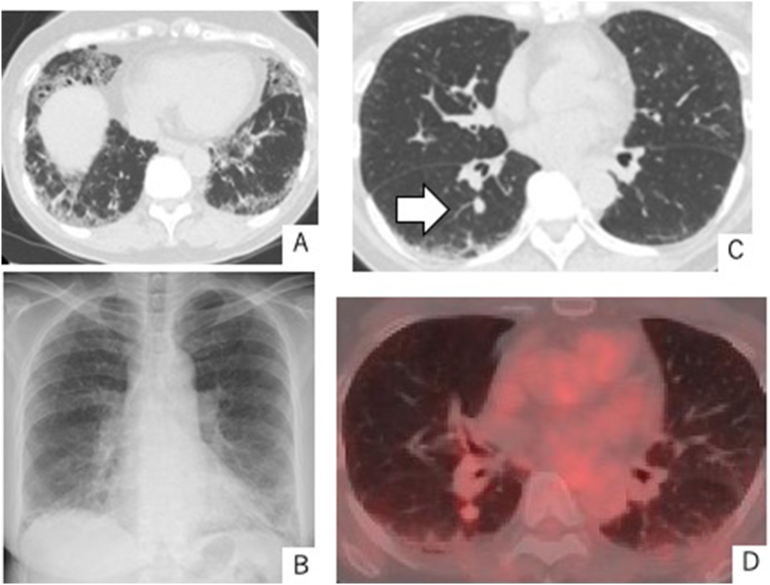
A: Chest CT reveals interstitial shadows, predominantly in bilateral lung bases. B: Chest radiography reveals no nodules. C: Chest CT reveals a 12 × 10 mm nodule (arrow) in the S6 segment of the right lower lobe. D: Accumulation of ^18^F-FDG is apparent in the area of nodular shadows on PET/CT.
